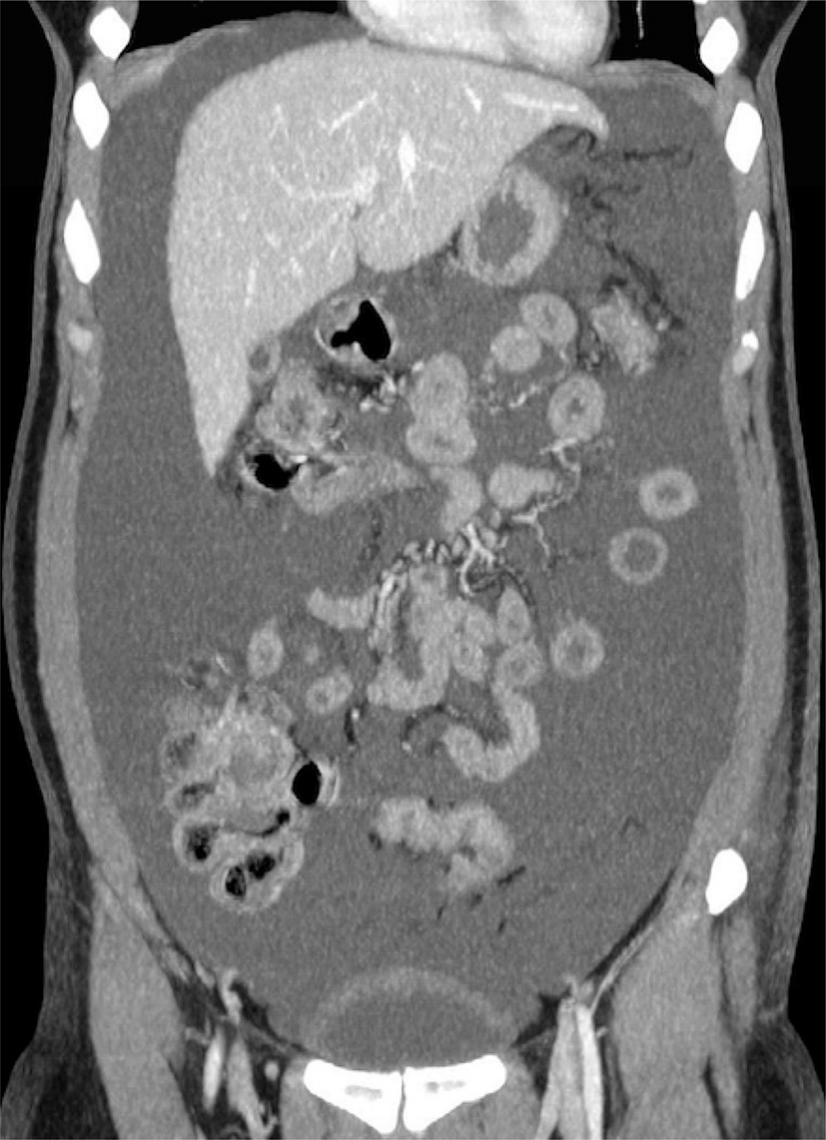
Coronal CT reformatted image showing widespread fluid within the abdomen.
